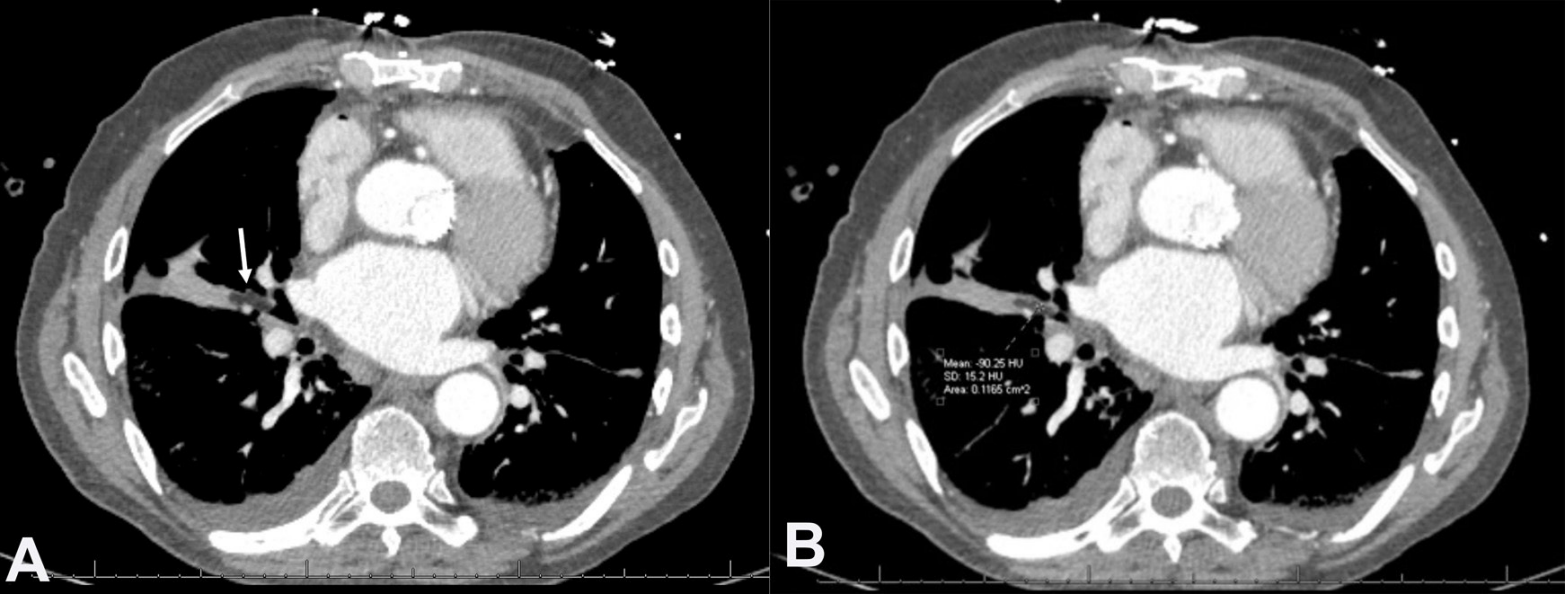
CT scan of the chest with IV contrast (A) Axial image shows a proximal right middle lobe bronchus hypodense lesion (arrow). (B) The lesion displays negative Hounsfield units (-90 HU) consistent with fat component.
